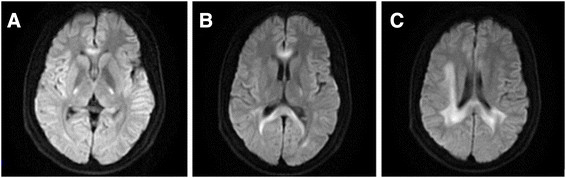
The second MRI after nine days of onset. Diffusion-weighted MRI afternine days of the onset of symptoms shows no change compared with the first MRI (A, B and C).
